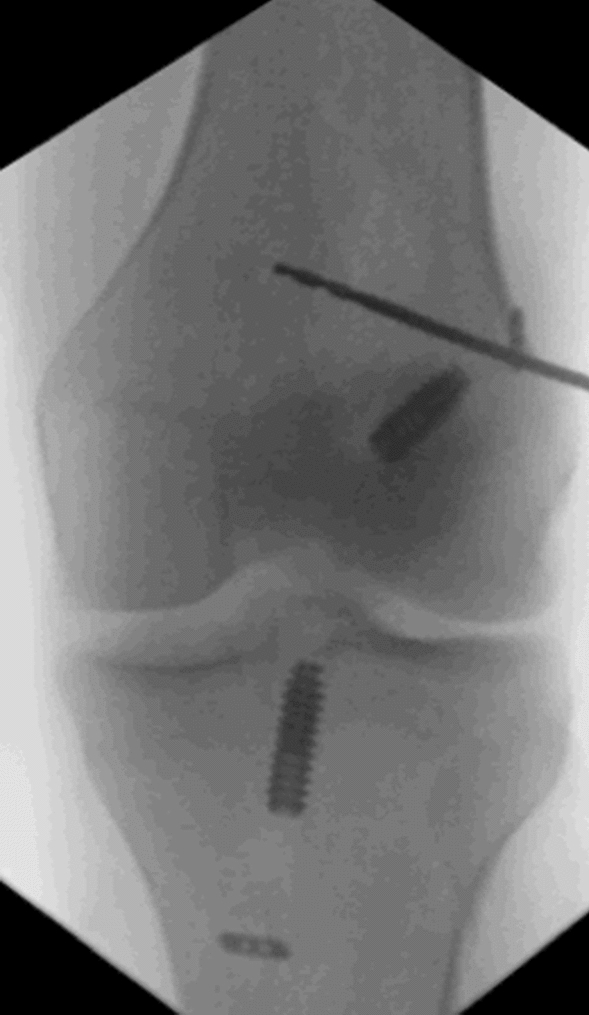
X-ray of the knee with the k-wire placed 1 cm proximal and posterior to the lateral epicondyle
